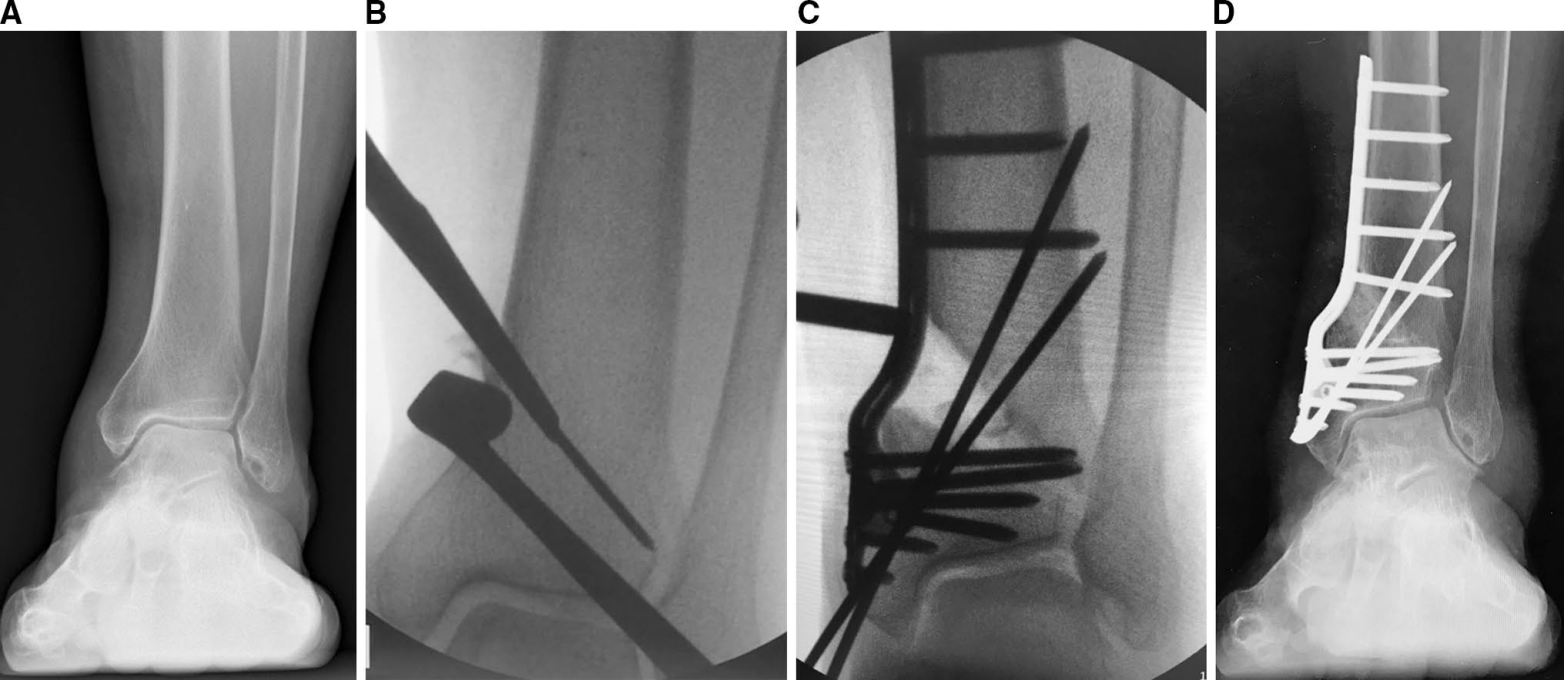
A 76-year-old female with stage 2 varus ankle osteoarthritis of her left ankle. She had bilateral total knee replacements. Weight-bearing radiographs before DTOO showed the narrowing of the joint space medially (a). The intra-operative radiograph shows the DTOO performed with a chisel (b). After separation of the osteotomy surfaces to the optimum degree, fixation was established with a curved plate (c). As ankle dorsiflexion after the osteotomy was −15°, the Achilles tendon was elongated (30 mm). The weight-bearing radiograph 1 year after DTOO shows the good preservation of ankle joint space with the sole of the hindfoot perpendicular to the axis of the tibia (d). The moderate instability of ankle joint present before DTOO has been improved and ankle joint pain resolved completely such that the patient could run. The range of motion was maintained after DTOO (dorsiflexion 5°—plantar flexion 20°)
